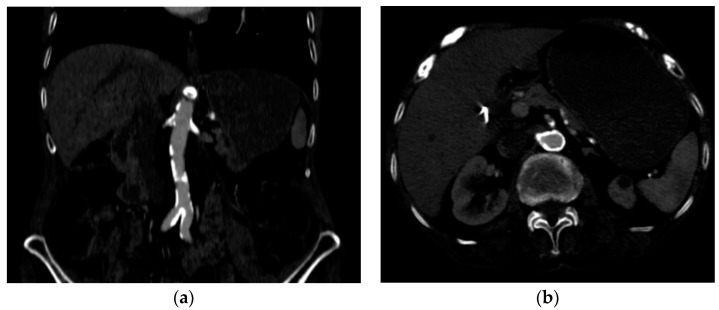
Computed tomography angiography (a) calcified atherosclerotic lesions on the aortic and iliac arteries; (b) circumferential, calcified lesions at the origin of the celiac trunk and superior mesenteric artery.
